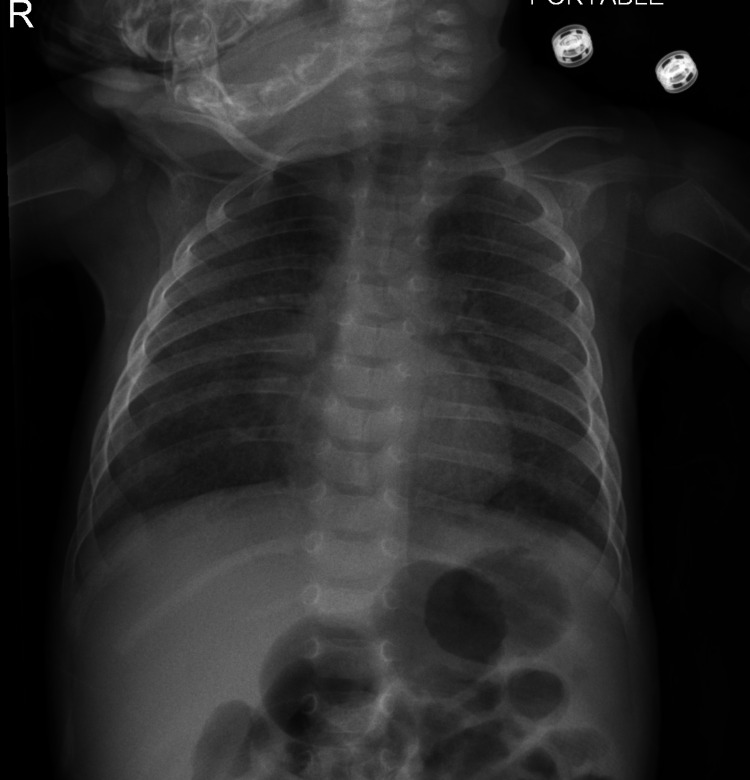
Chest X-ray revealed bilateral opacities at mid and lower lung zones.
